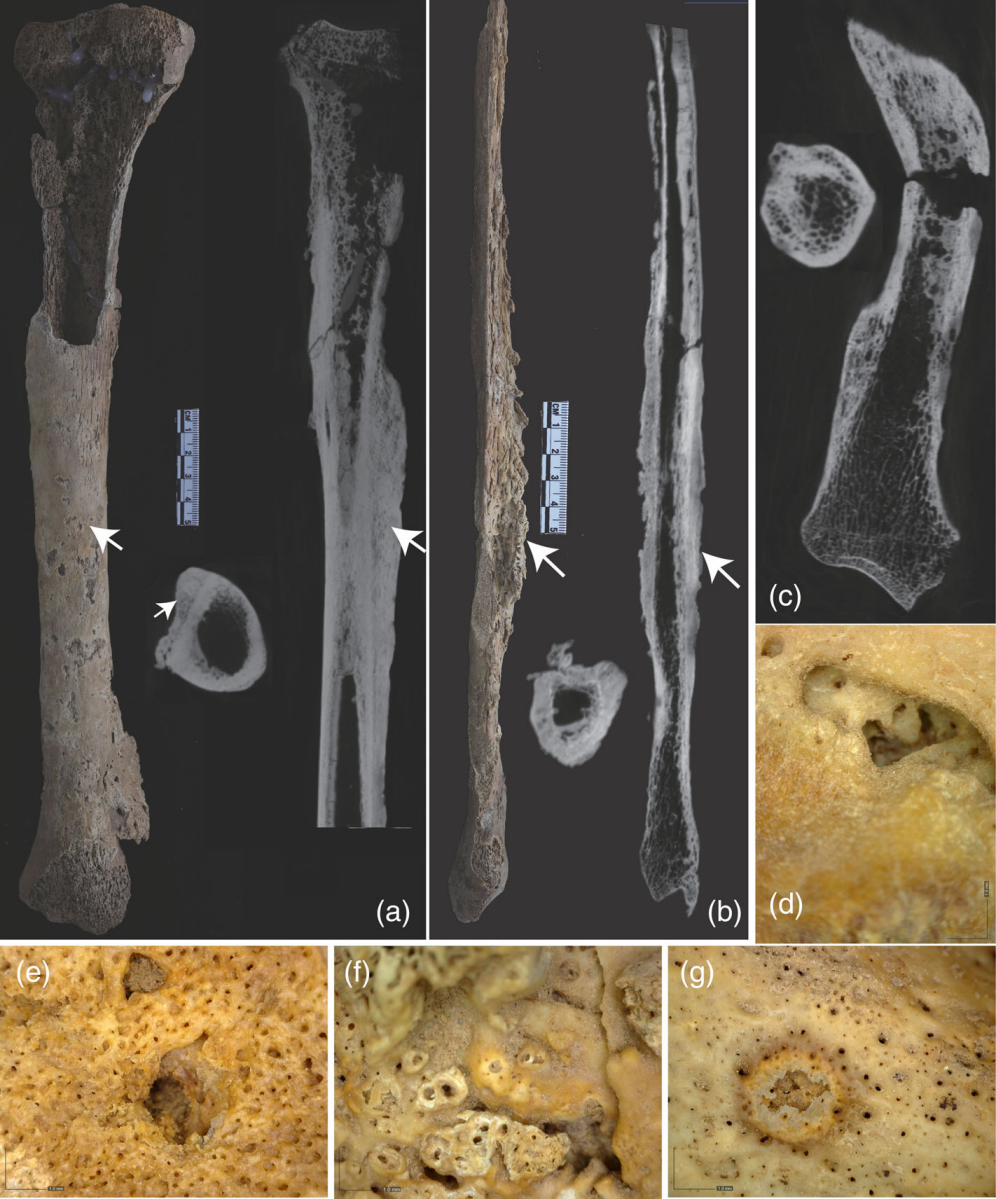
Pathological changes on the male skeleton from Xingfulindai burial M173: (a) The right tibia and CT slices of the right tibia, notice the visibility of original cortex and endosteal bone formation; (b) Florid periosteal reaction on the right fibula and CT slices of right fibula; (c) CT image of the right radius, (d) Superficial lesion on the right radius at 50× magnification; (e–g) Superficial lesion on the right tibia at 50× magnification
